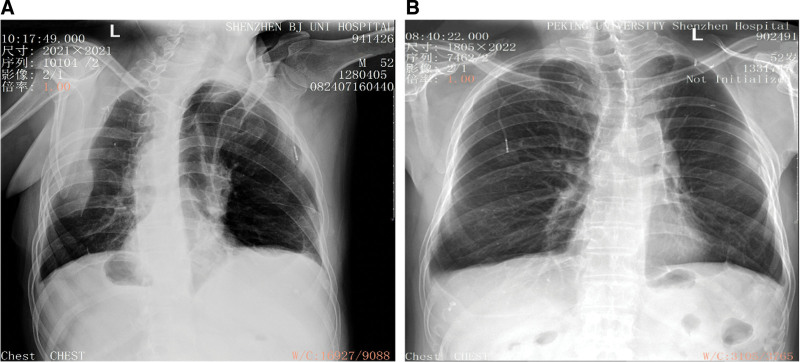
(A) Collapse of the upper left chest suggests a dislocation of the left scapula into the left pleural cavity. (B) After closed reduction of the scapular prolapse, we can see that the left scapula has been restorated on the chest radiography.
